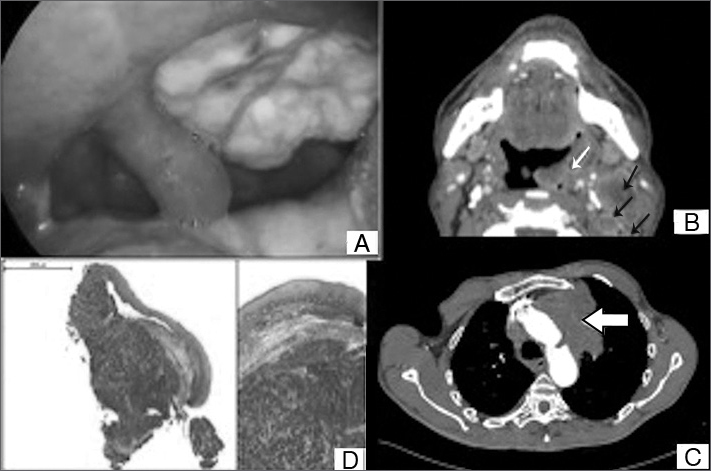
A: macroscopic view of a tumor in the left tonsillar bed; B: axial view of a neck CT scan (white arrow: tumor in the oropharynx; black arrows: lymph nodes); C: axial view of a chest CT scan (white arrow: tumor in the left upper lobe); D: squamous mucosa invaded by small-cell lung carcinoma characterized by mid-size trabecular tumor cells with round and oval nuclei and scarce cytoplasm; original magnification and 35x original magnification.
